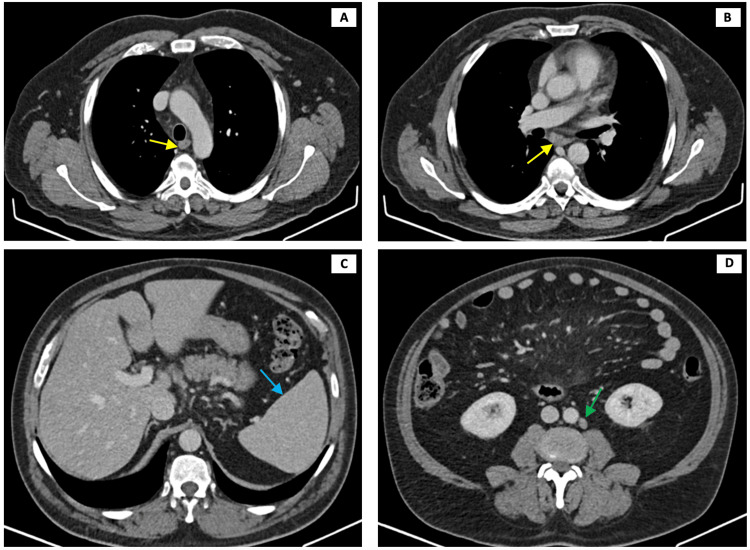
Thoracoabdominal CT scan showing mediastinal lymphadenopathy (A and B, yellow arrows), slight splenomegaly (C, blue arrow), and enlarged retroperitoneal lymph nodes (D, green arrow) CT: computed tomography
